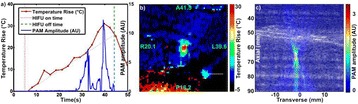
a Passive acoustic mapping (PAM) and MR-derived temperature rise over time. b MRI temperature map and (c) acoustic map for cavitation-enhanced focal heating
